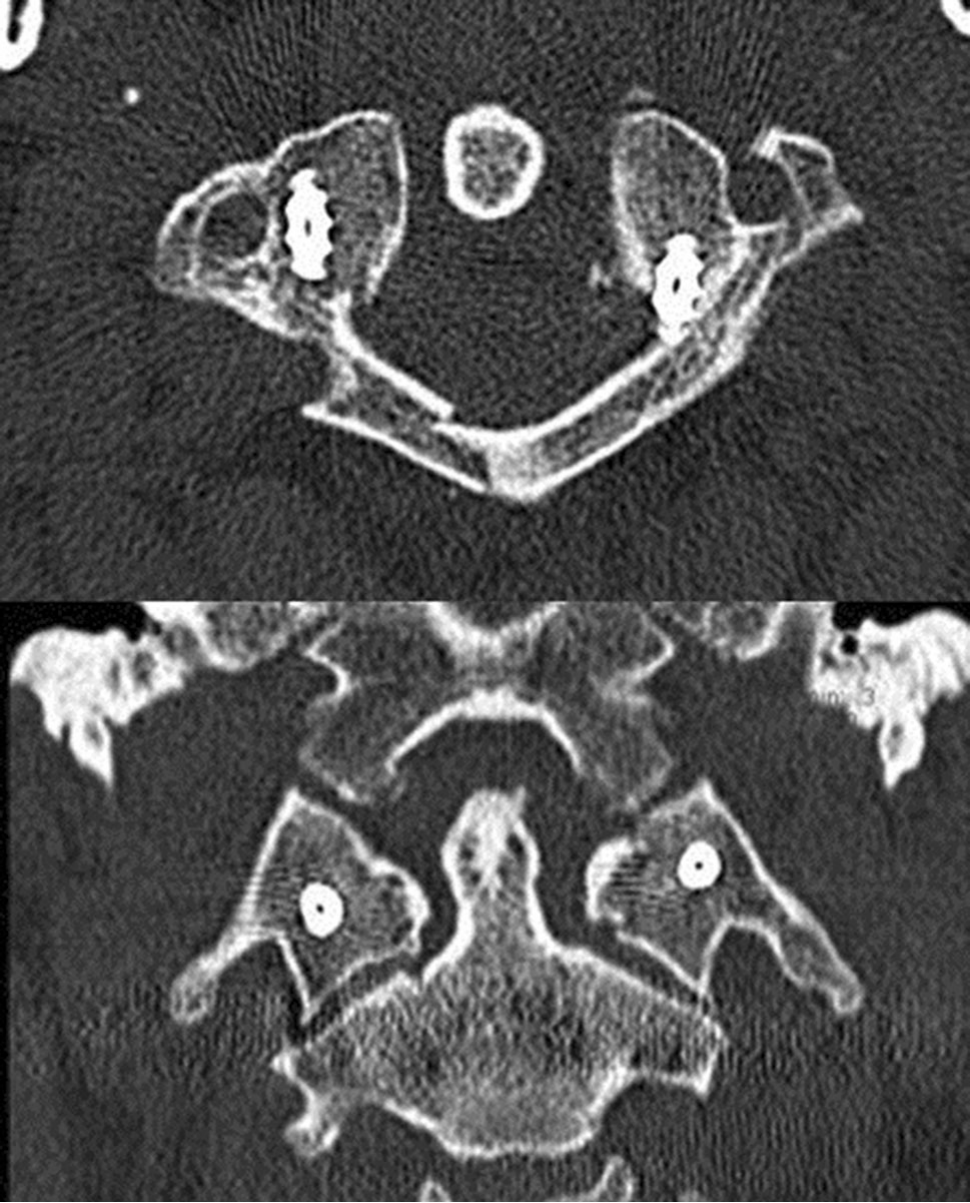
Transversal (*top*) and coronal (*bottom*) CT images of patient 3 after surgery: good reduction of the left lateral mass and closure of the arches
